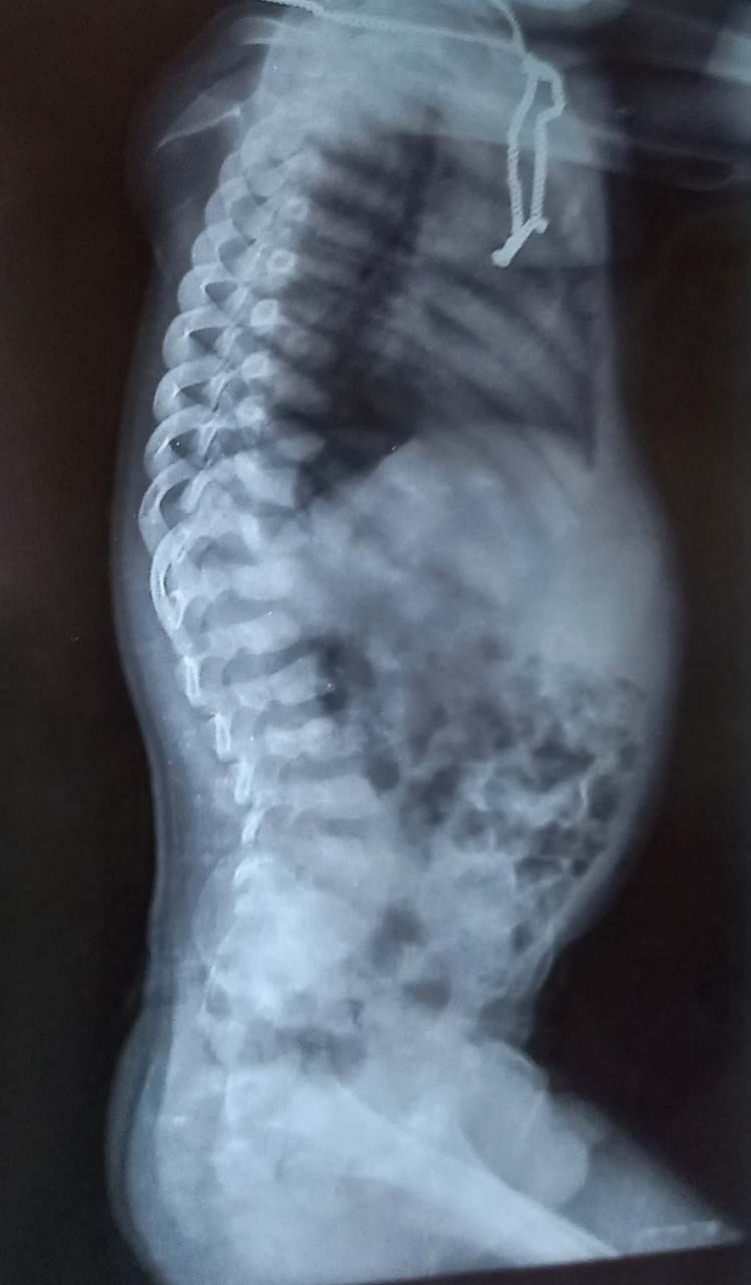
X-ray spine lateral view showing beaking in the thoracolumbar region.
